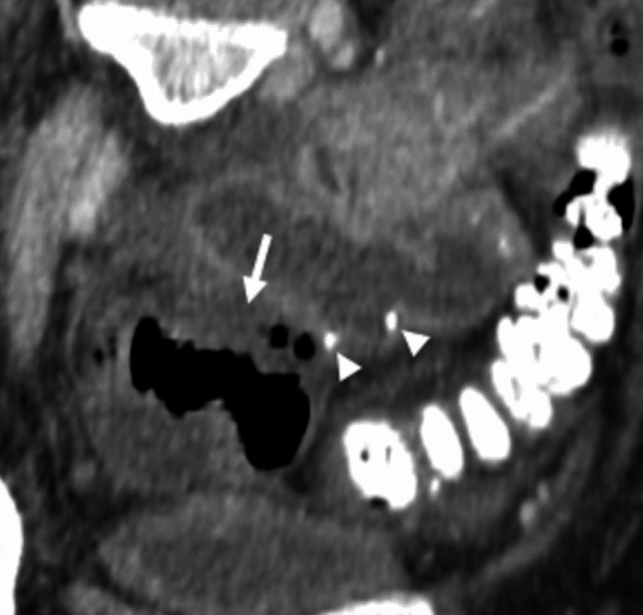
Doudenoenteric anastomotic leak. A 52-year-old male with a history of type 1 diabetes mellitus underwent pancreas after kidney transplantation, presented with abdominal pain and an elevated white blood cell count. Contrast-enhanced computed tomography demonstrates a nearly well-defined non-enhancing fluid collection (arrow) with foci of air adjacent to the doudenoenteric anastomosis (arrowhead) secondary to enteric leak. The fluid collection was treated with percutaneous drain placement
